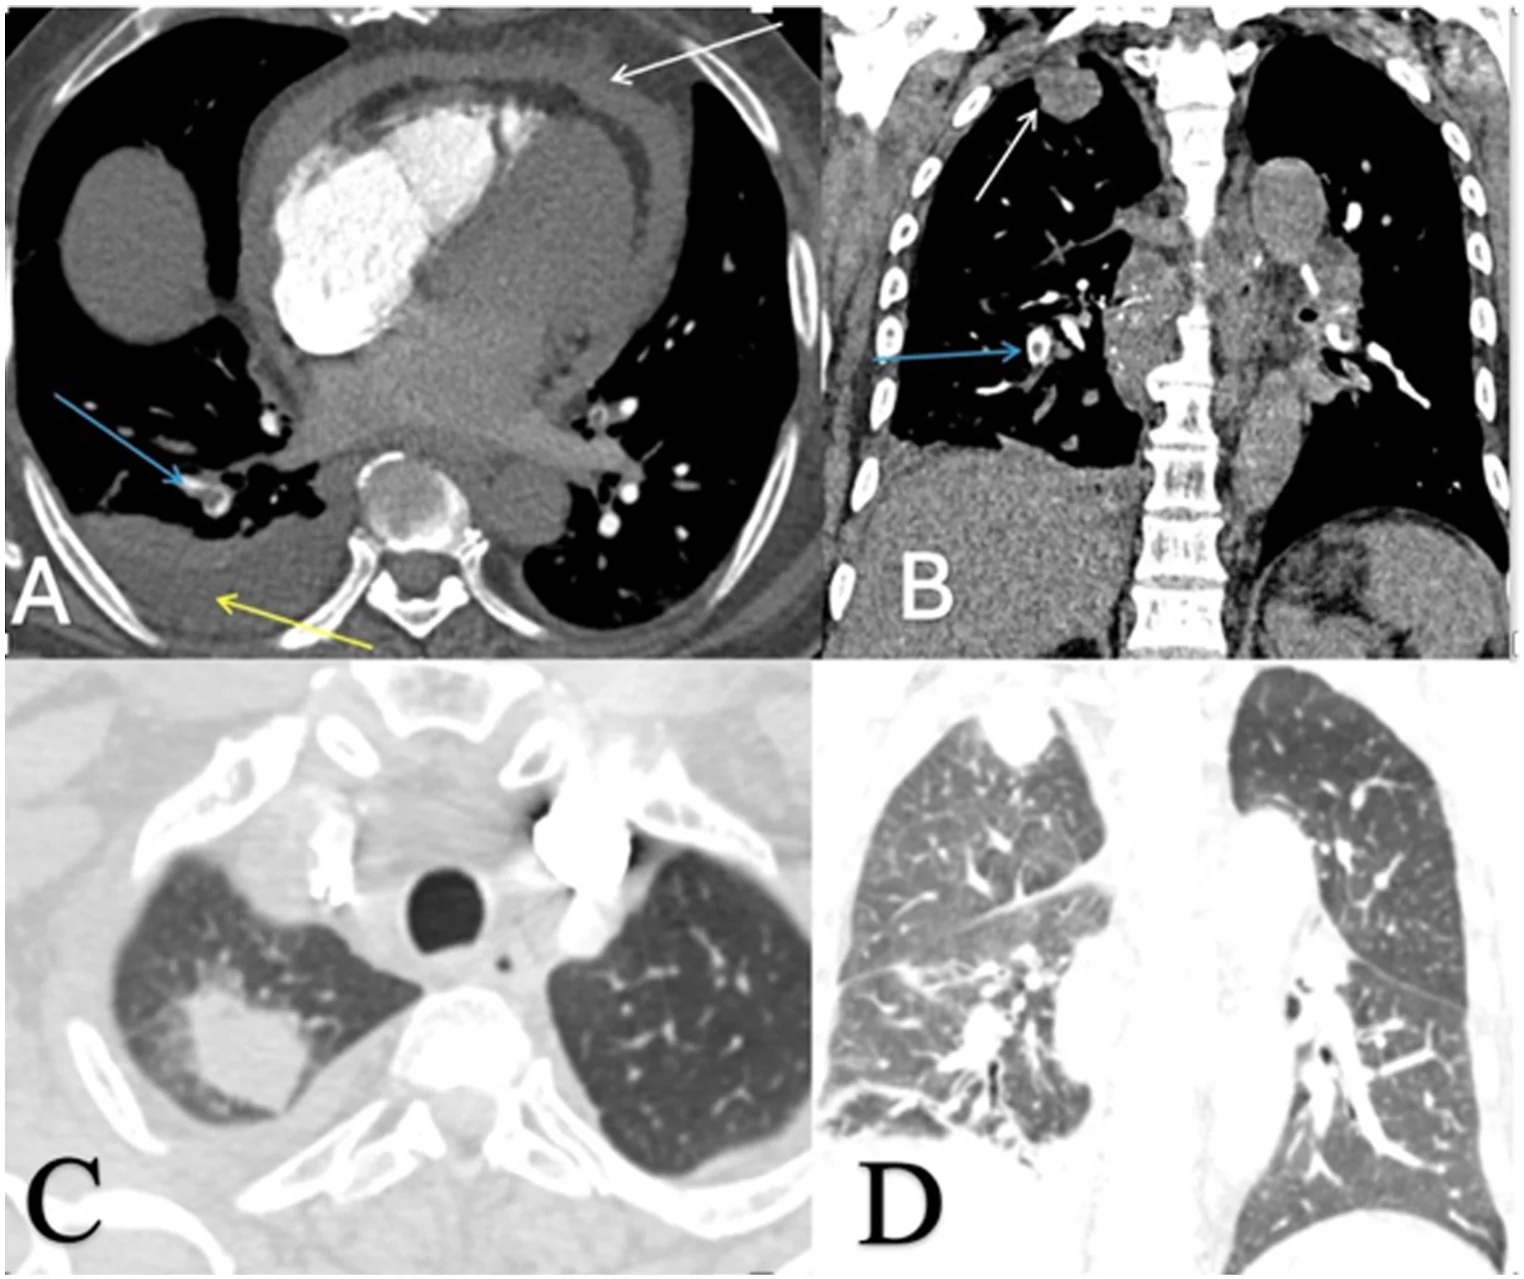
Serial imaging demonstrating disease progression 6 months apart. Initial contrast-enhanced CT (3 mm reconstructions): 1A: Axial reconstruction showing circumferential visceral and parietal pericardial thickening with associated pericardial effusion (white arrow). Right-sided pleural effusion with adjacent passive atelectasis (yellow arrow). Filling defects within the pulmonary arteries consistent with pulmonary emboli (blue arrow). 1B: Coronal reconstruction showing well-circumscribed right upper lobe apical nodule (2.1 cm × 1.4 cm × 1.3 cm) (white arrow). Calcified hilar and paratracheal lymph nodes suggesting prior granulomatous disease. 1C (axial) and 1D (coronal): 3 mm axial reconstruction clear of active pulmonary TB.
